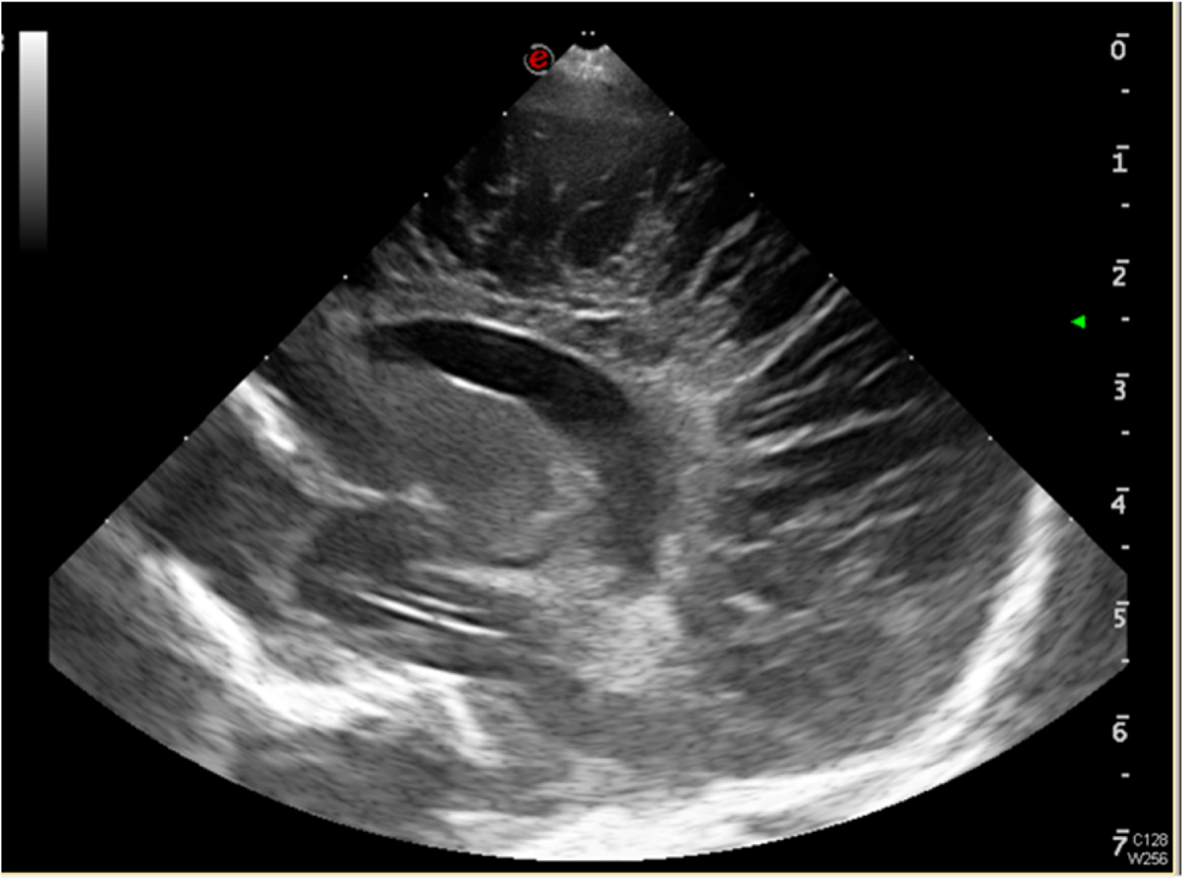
Postnatal transfontanelar ultrasound of second baby at twenty-second day of life showing multiple cystic cavities in bilateral periventricular regions
